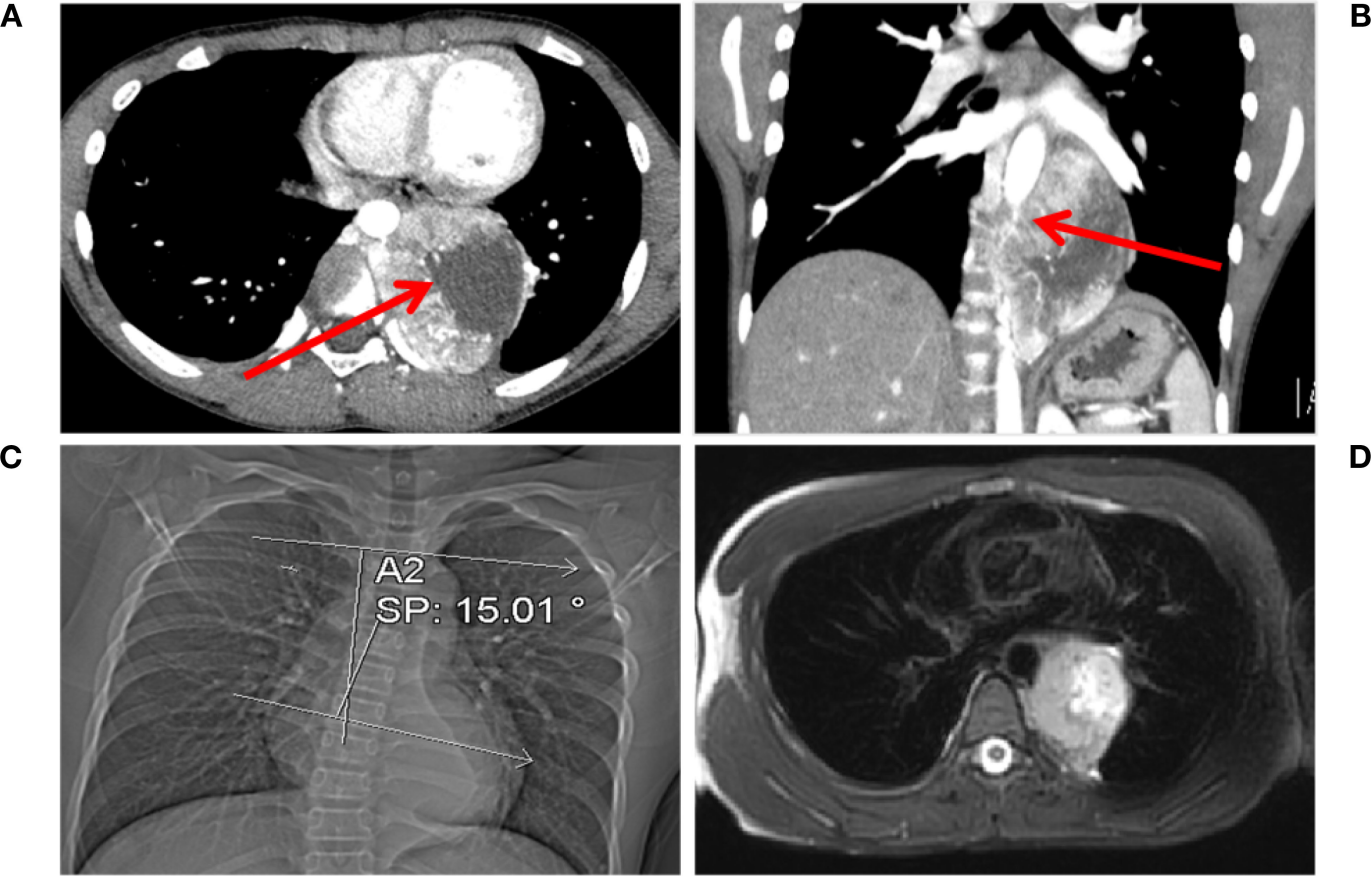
Chest CT and MRI findings. (A) Multiple vascular shadows are visible in the arterial phase, accompanied by an irregularly enhanced mass. Necrosis is present within the tumor, as indicated by the red arrow. (B) The tumor encircles the aorta (indicated by the red arrow) in the coronal view. (C) The tumor compresses the spine, causing scoliosis (Cobb angle: 15.01°). (D) T2-hyperintense lesion with clear margins is observed, with no involvement of the intervertebral foramen or spinal cord.
